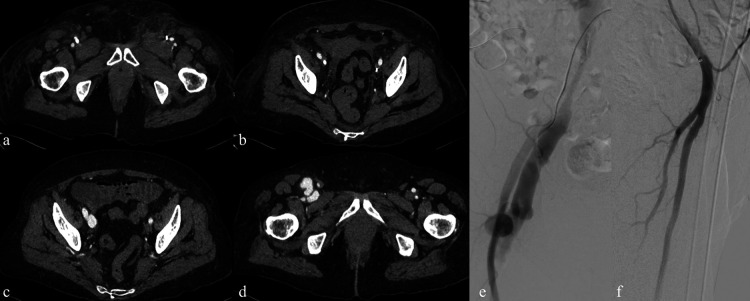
Imaging findings of a vascular complication related to temporary femoral central venous catheter placement for dialysis. (a,b) Contrast-enhanced CT showing a temporary dialysis catheter correctly positioned within the iliac vein but with inadvertent passage into the superficial femoral artery (circle). (c,d) Follow-up CT after catheter removal demonstrating the development of a pseudoaneurysm (arrow) and an arteriovenous fistula. (e,f) Digital subtraction angiography confirming the vascular injury and subsequent treatment with covered stent placement.
